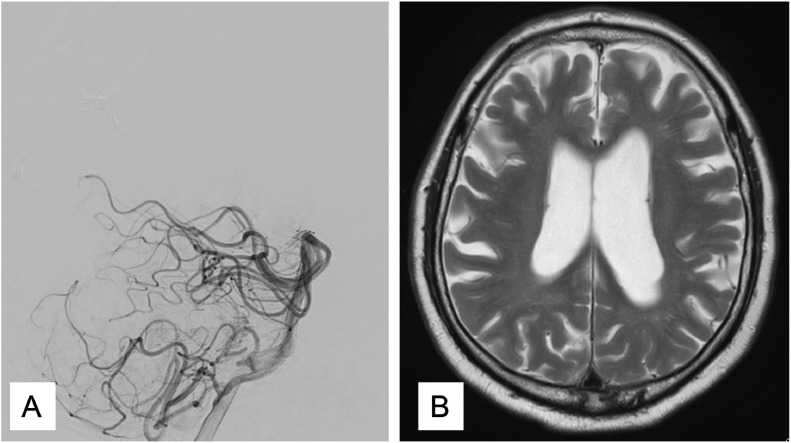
T2-weighted imaging showing no abnormal vessels (A). The lateral view of the left vertebral angiography showing no recurrence of the falx dural arteriovenous fistula (B)
